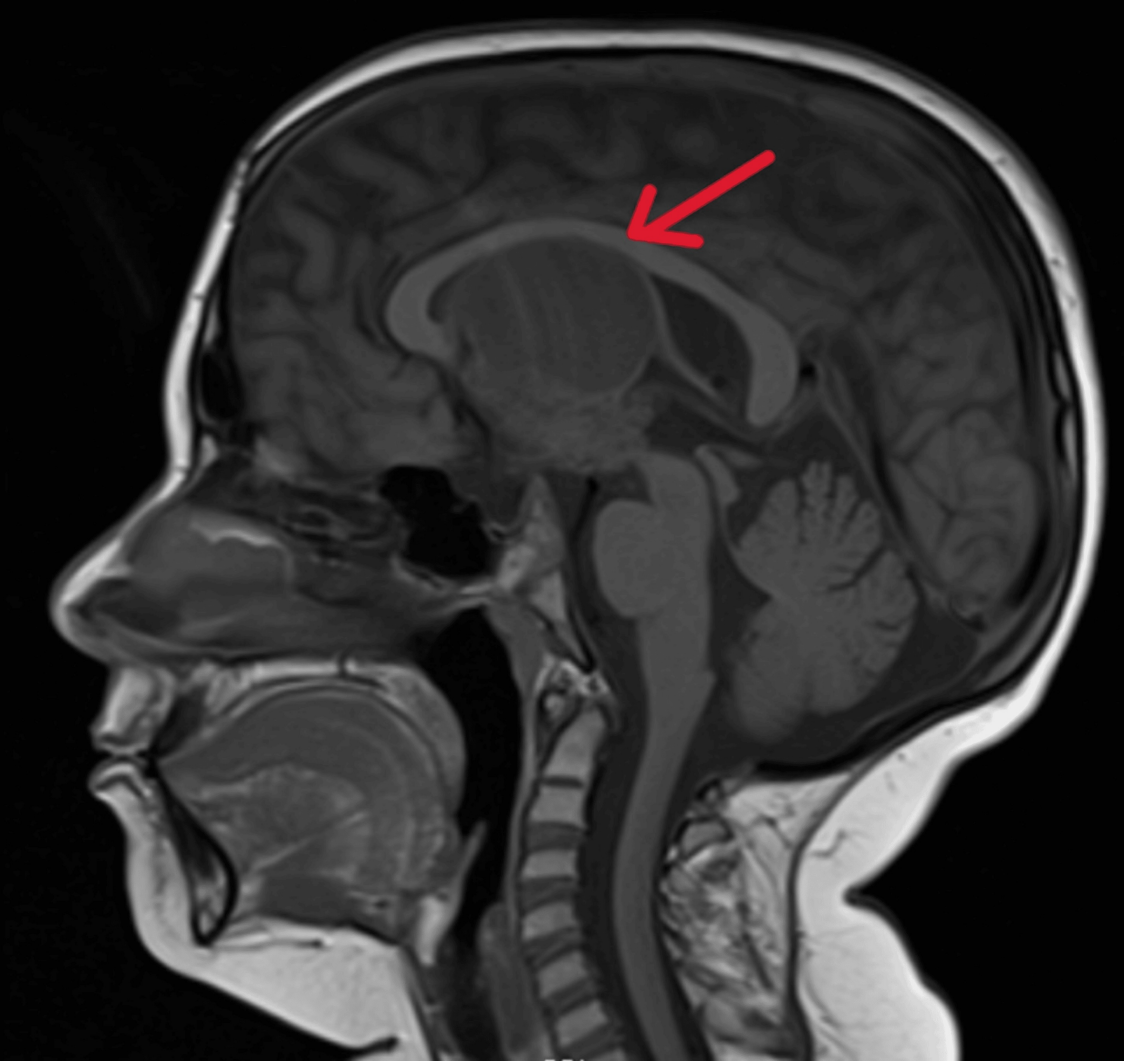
MRI brain with and without contrast, 2023 The arrow depicts craniopharyngioma with a large midline cyst extending into the ventricles which measured approximately 5.5 x 3.5 x 3.5 cm and was almost double compared to the preoperative MRI from September 2022. Two paramedian cysts also significantly increased in size, measuring approximately 1.5 cm each.
